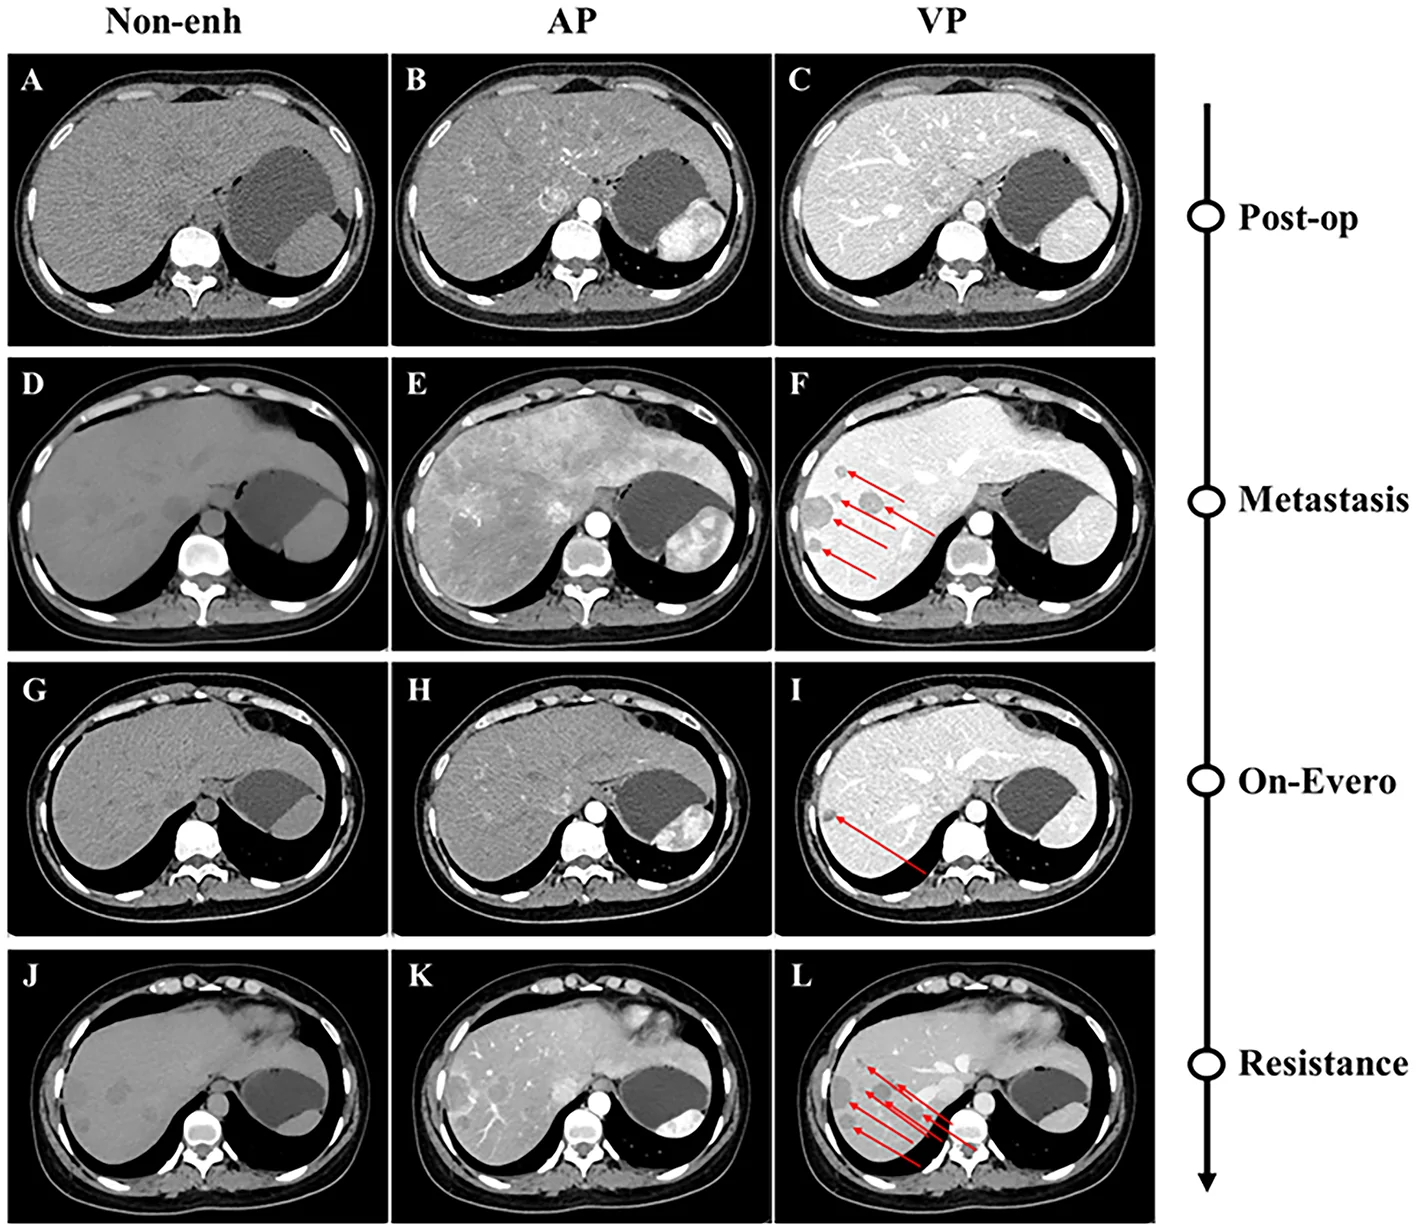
Serial hepatic imaging demonstrates the clinical course from metastatic disease to treatment response and subsequent acquired resistance. (A–C) Baseline contrast-enhanced CT images of the liver prior to the development of metastases. (D–F) Surveillance CT at 10 months post-surgery showing new hypodense hepatic metastatic lesions (arrows). (G–I) Follow-up CT after initiation of everolimus, demonstrating complete radiographic resolution of metastatic lesions. (J–L) CT imaging at 16 months of therapy showing disease progression with new hepatic metastases (arrows), consistent with acquired resistance. AP, arterial phase; VP, venous phase; Non-enh, non-enhanced.
